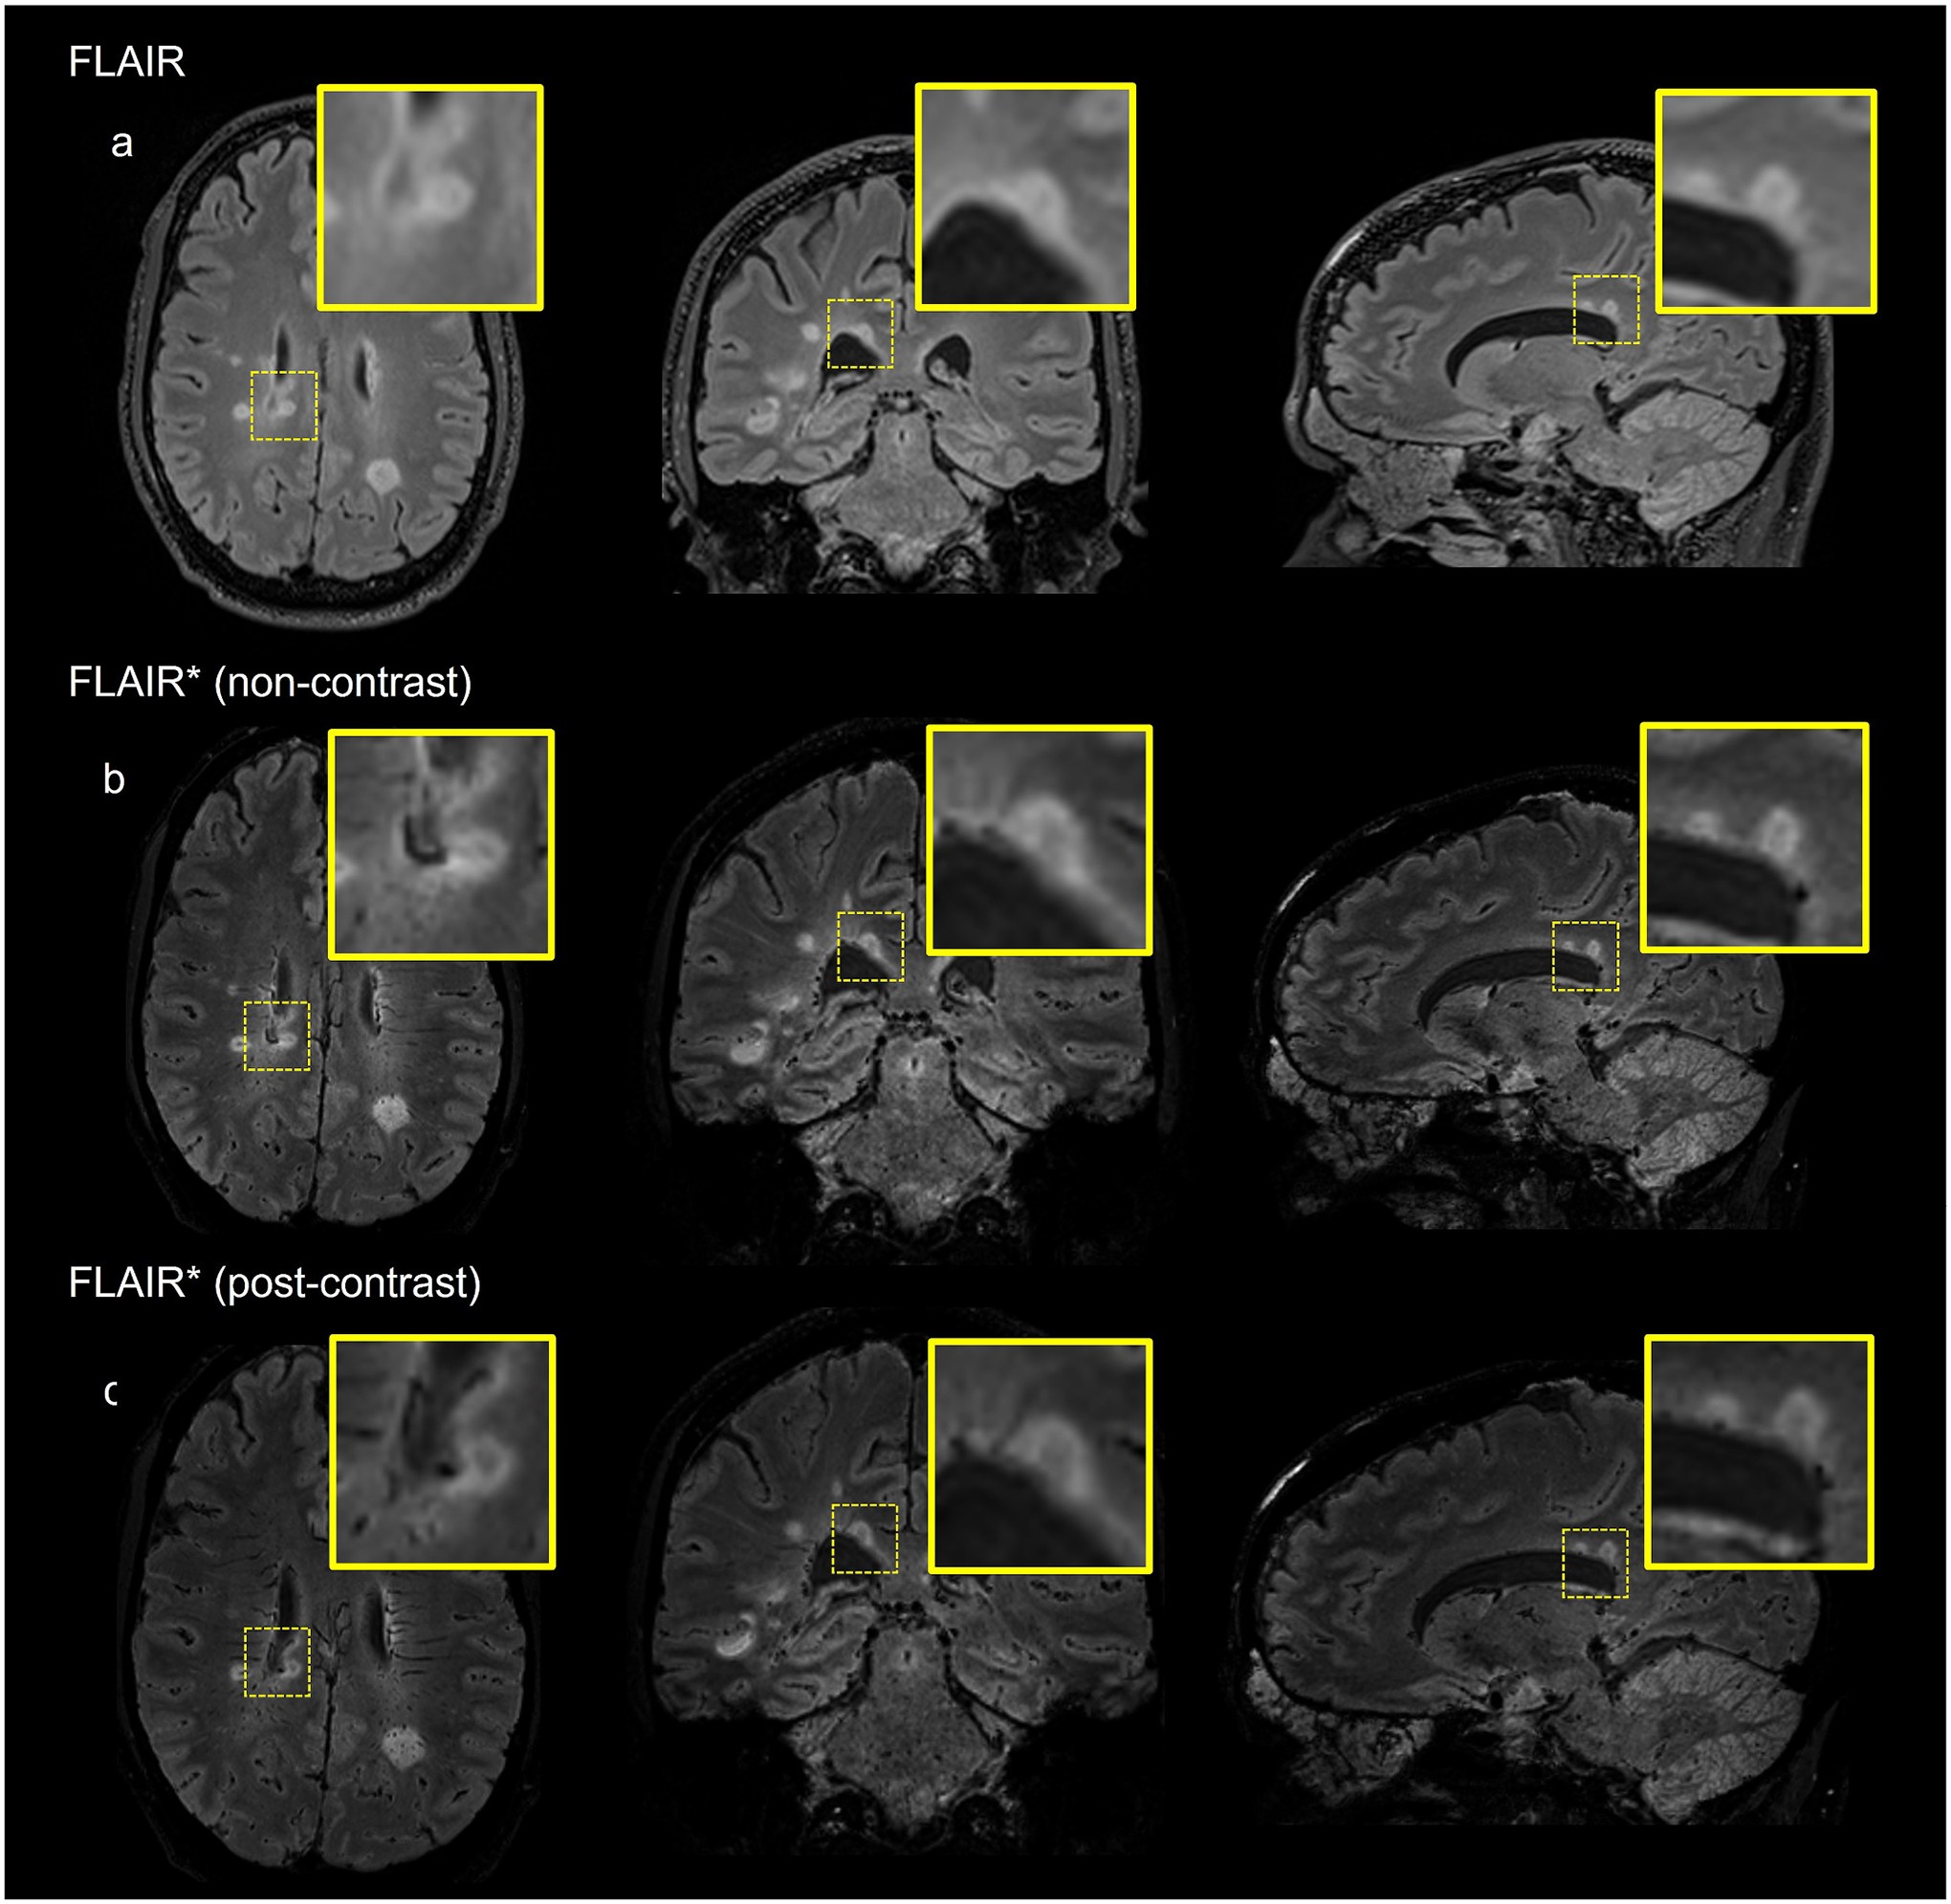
Example of a central FLAIR hypointensity in three planes (a), consistent with CVS on non-contrast (b) and post-contrast FLAIR* (c).
